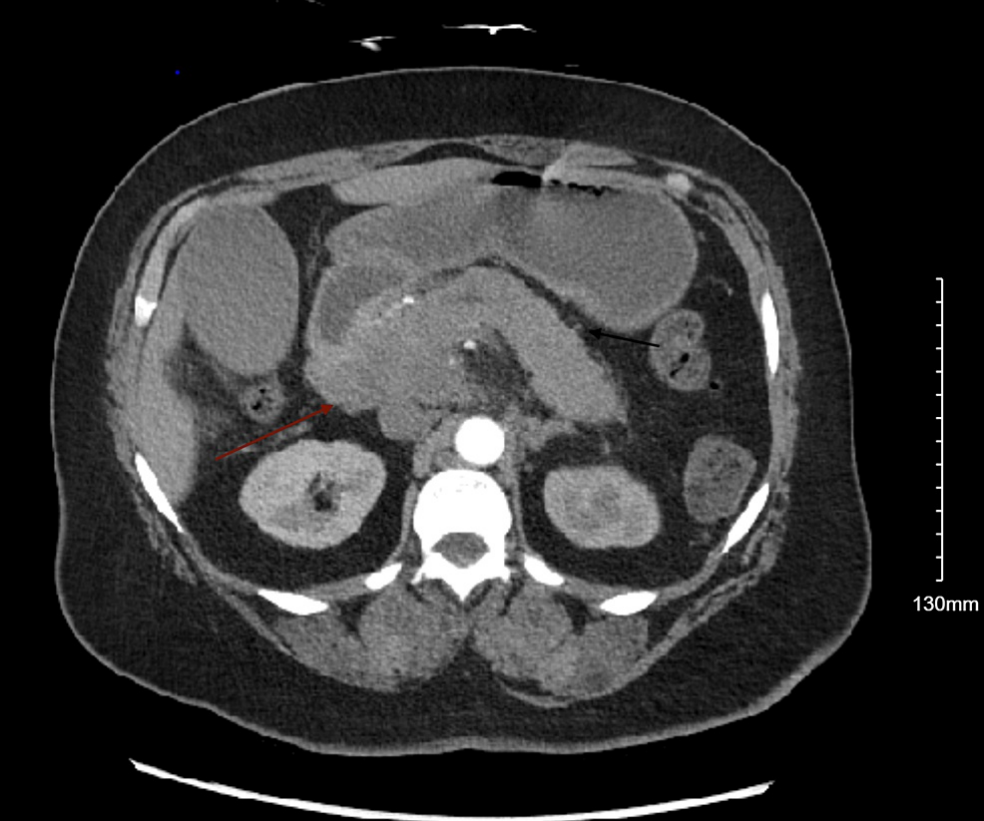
CT of the abdomen. Red arrow: pancreas head. There are diffuse thickening of the pancreas and significant surrounding fat stranding (black arrow), especially in the region of the pancreatic body, which is concerning for pancreatitis.
